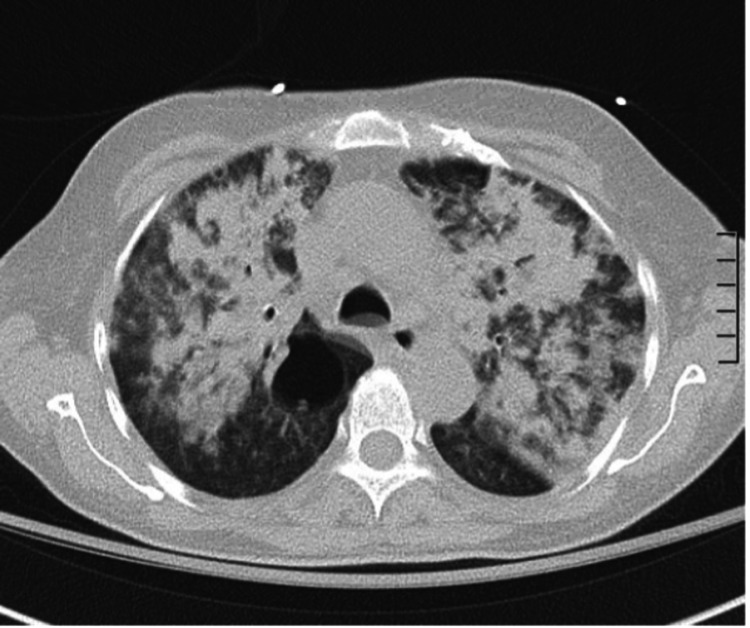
Computed tomography scan of the chest showed diffuse airspace opacities within the lungs bilaterally
